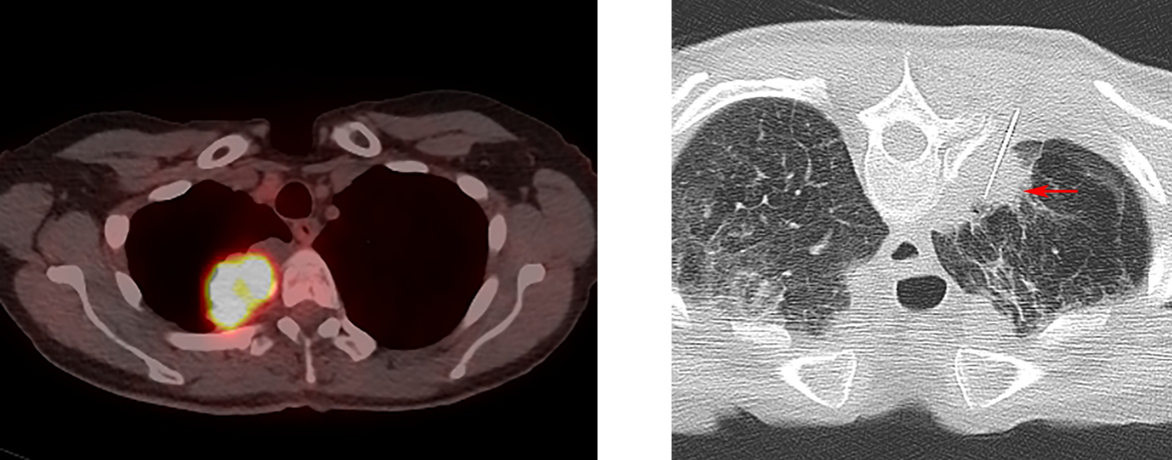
Example of likelihood of adequacy score 3 (high). (A) PET/CT showing right lung lesion that is large and FDG avid. Also, there is no aerated lung in the planned biopsy path. (B) Arrow points to needle in right lung mass.
